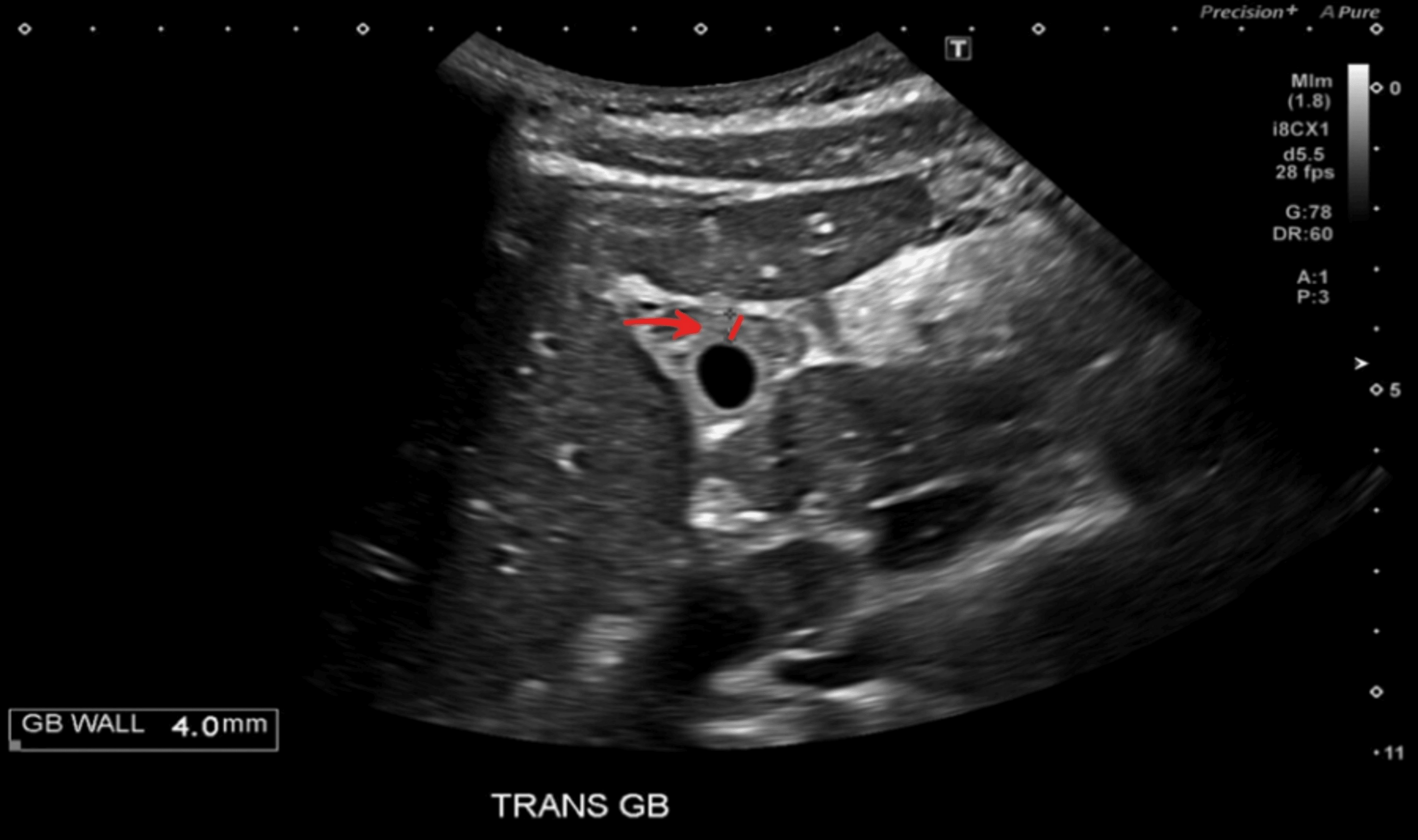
Abdominal ultrasound demonstrating increased gallbladder wall thickness at 4.0 mm Red arrow pointing to the thickened gallbladder wall (solid red line).
